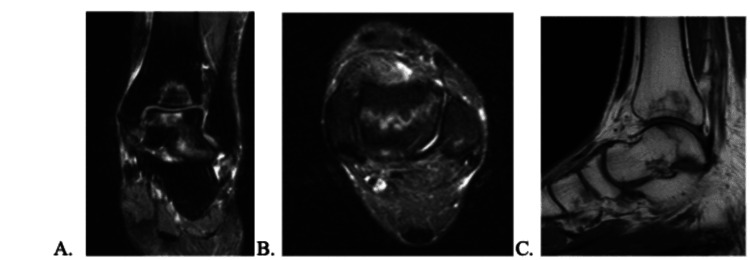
MRI that initially showed AVN on April 15, 2022. In addition, there is a nondisplaced fracture of the talar body. AVN: avascular necrosis
